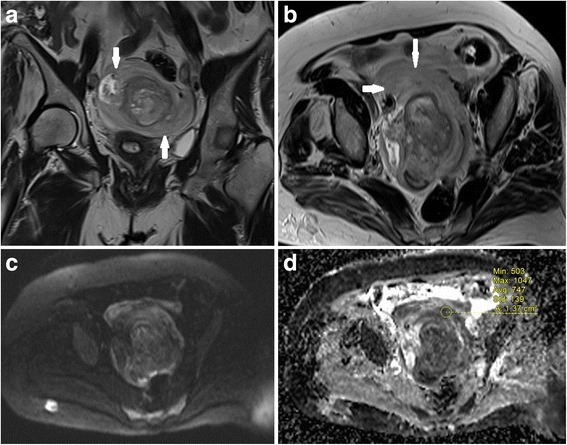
A female patient with left lower quadrant pain for 4 days. She was proved to have haemorrhagic ovary torsion due to granulosa cell tumour. a T2-weighted coronal MRI reveals an enlarged and oedematous left ovary (arrows) with an acompanying central mass. b T2-weighted axial MRI shows twisted pedicle sign (arrows) and central mass. c b 1000 image shows diffuse hypointensity of the enlarged ovary. d ADC map shows diffusion restriction of the peripheral stroma of ovary (ADC value, 0.74 × 10^−3^ mm^2^/s)
